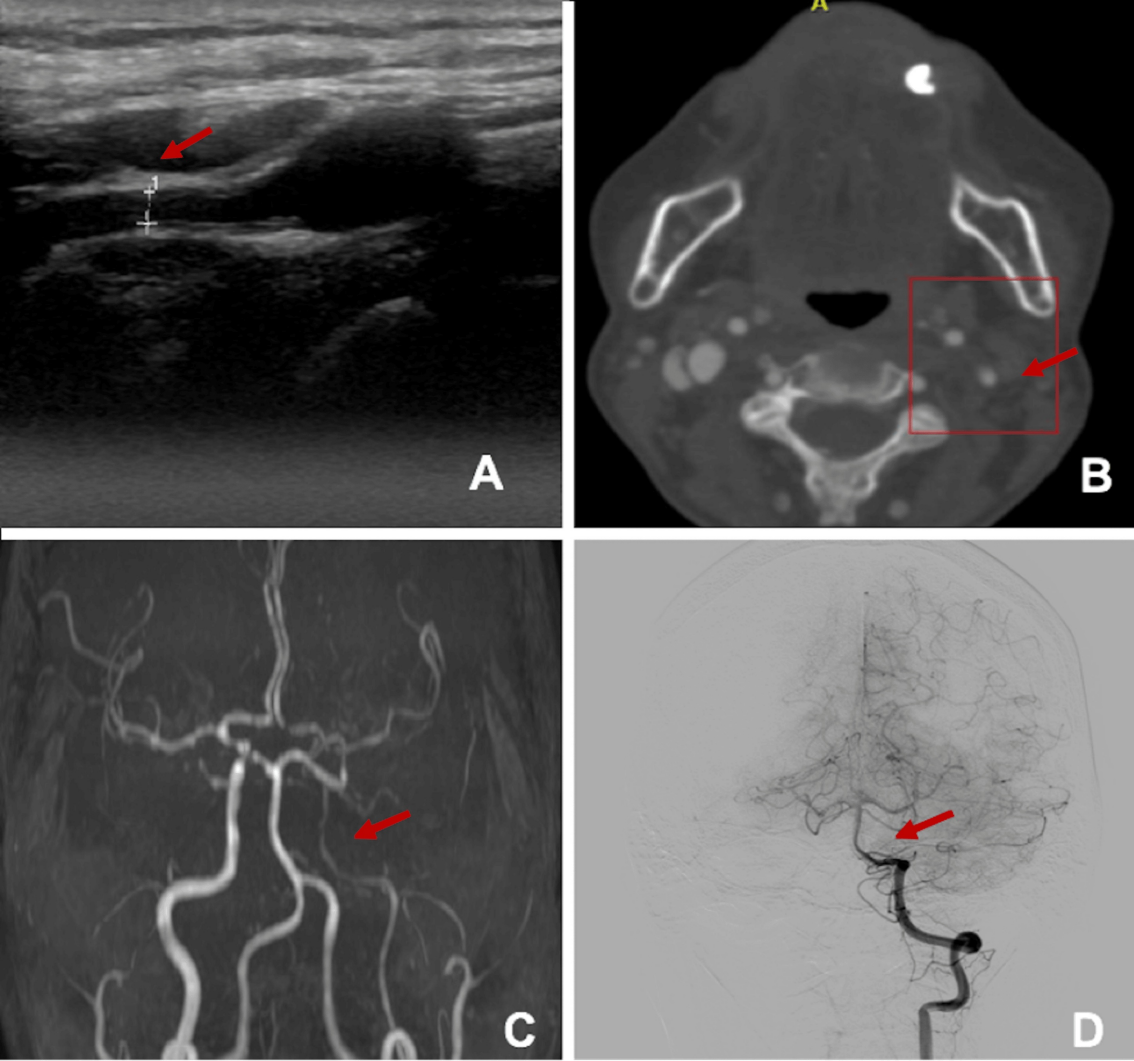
Reduction in the left internal carotid artery caliber in its cervical segment in (A) carotid Doppler ultrasonography and (B) neck CT angiography (red arrow), and with filiform discharge distal in its supraclinoid segments in (C) cranial MR angiography and (D) cerebral pan-angiography (red arrow) MR: magnetic resonance
